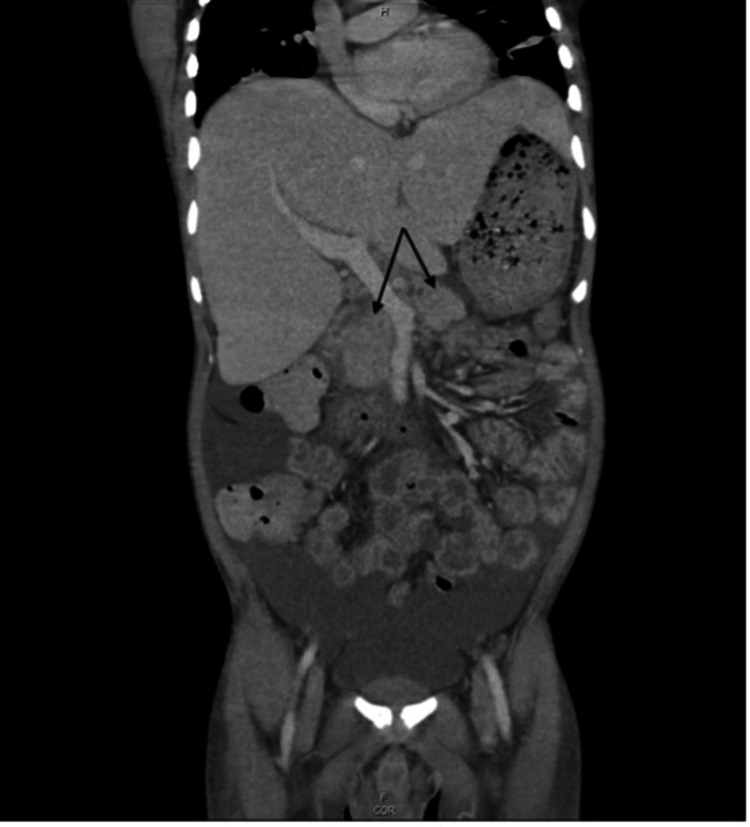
CT of the abdomen and pelvis with contrast, coronal view There is diffuse ascites throughout the abdomen. Arrows from left to right indicate necrotic para-aortic and celiac lymph nodes.
